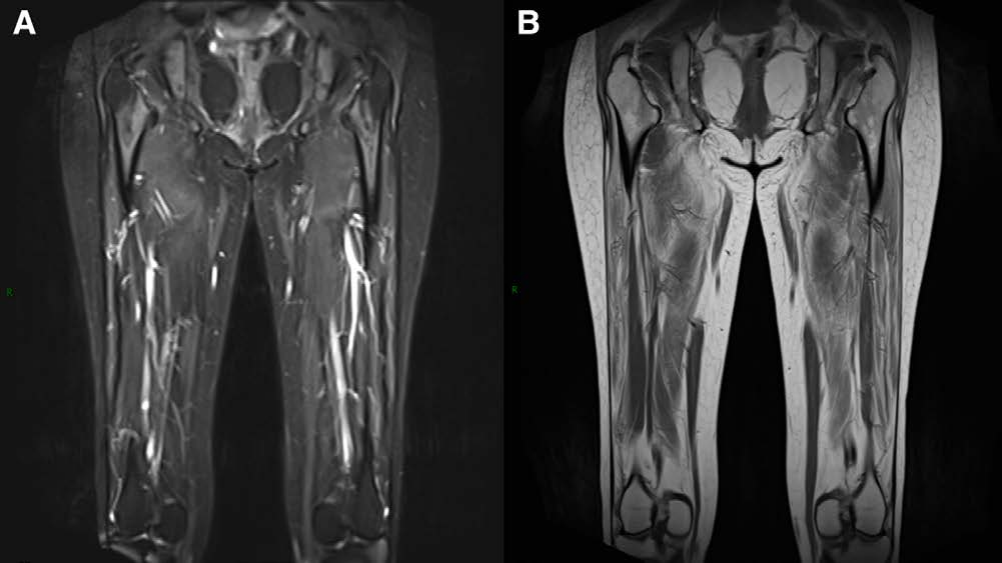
MRI results of the patient’s bilateral thighs. The shape of the bilateral femurs was normal on MRI, and an uneven T2 fat-suppressed high signal consistent with edema was seen in the upper medullary cavity with unclear margins (A). Bilateral long T2WI signal intensity suggestive of myopathic changes was observed in the lower segment of the vastus medialis muscles (B). MRI = magnetic resonance imaging.
